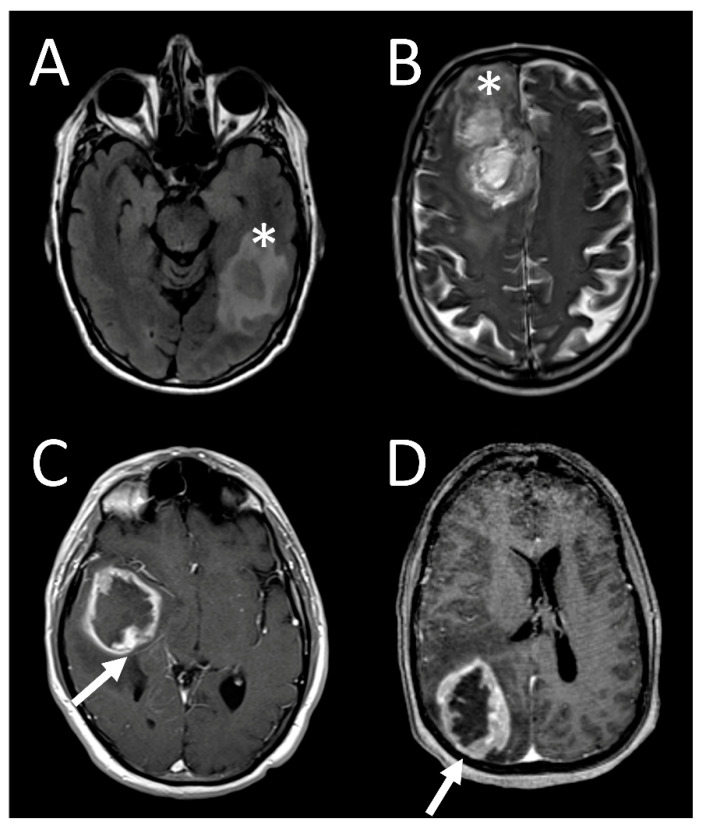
Sample imaging features of glioblastoma without 19/20 co-gain. FLAIR image of *MGMT* methylated tumor located in the left temporal lobe (A, asterisk). T2 images of *MGMT* unmethylated tumor located in the right frontal lobe (B, asterisk). T1 postcontrast image of *MGMT* unmethylated tumor located in the right temporal lobe demonstrating ependymal extension (C, arrow). T1 postcontrast image of *MGMT* unmethylated tumor located in the right parietal lobe demonstrating pial invasion posteriorly (D, arrow).
